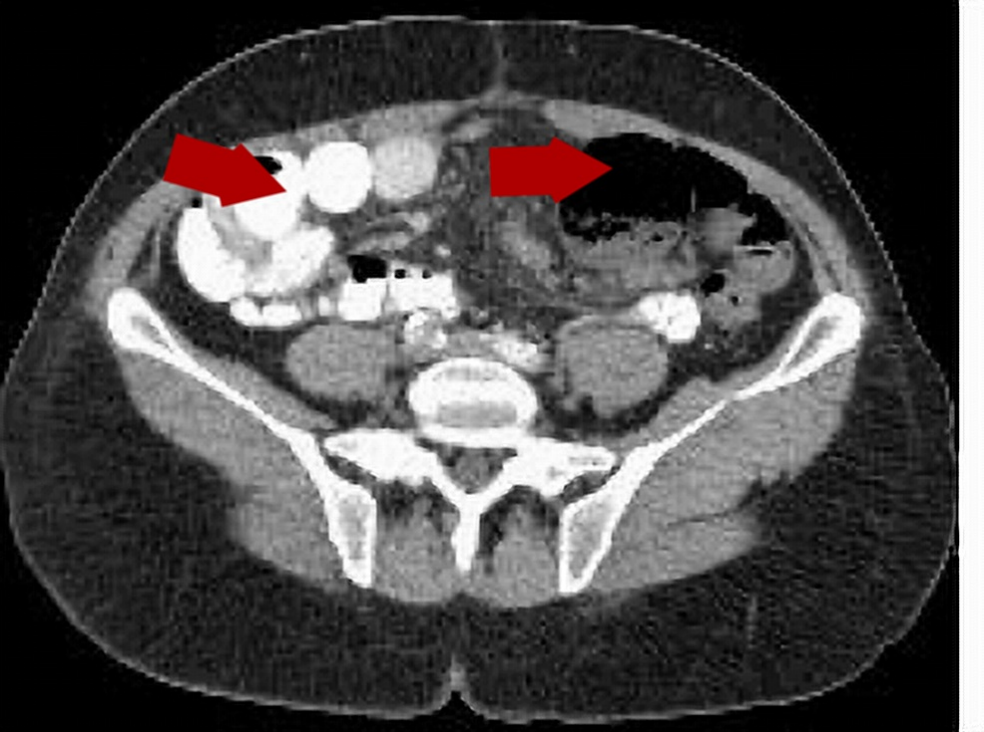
CT scan of abdomen and pelvis revealed focal dilation of mid-jejunal small bowel with inflammatory changes in the mesenteric fat and a jejunal diverticulum.
